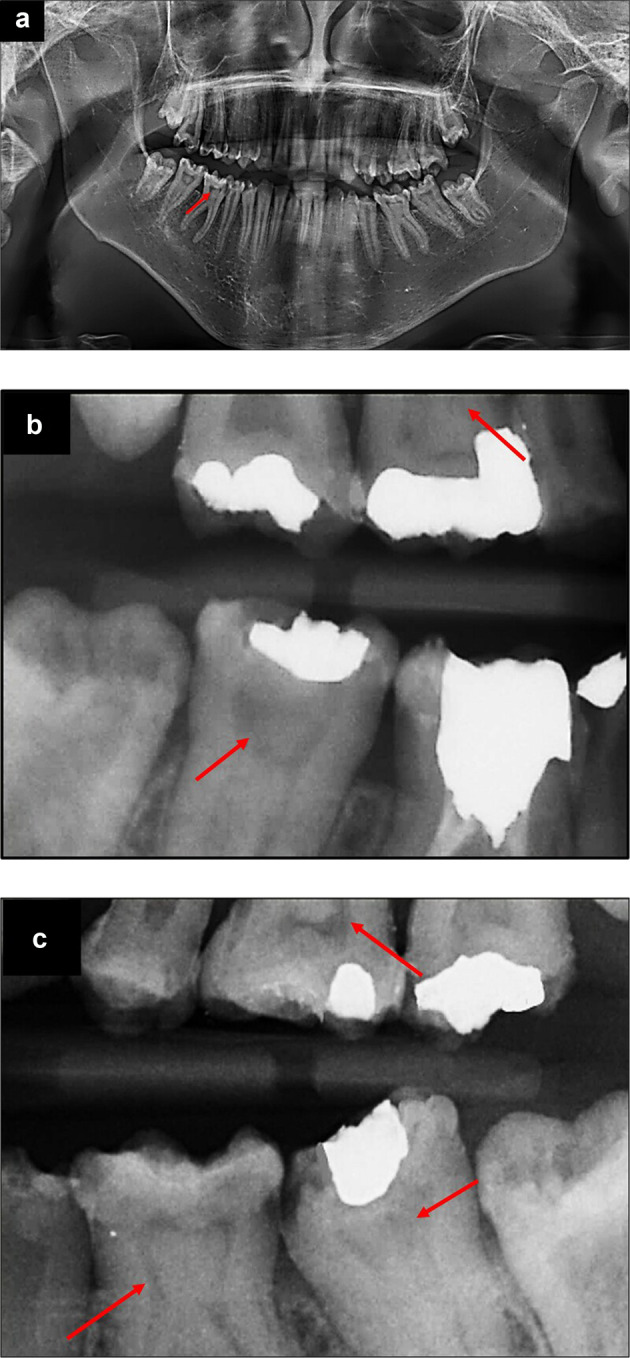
Dental radiography: Orthopantomogram and bitewing radiographs. Orthopantomogram (a) *showing* taurodontism in all upper, lower second and third molars with curved roots in lower first molars. Right (b) and left (c) bitewings showing pulp stones (red arrows) in all first molars and lower right, left second molars.
